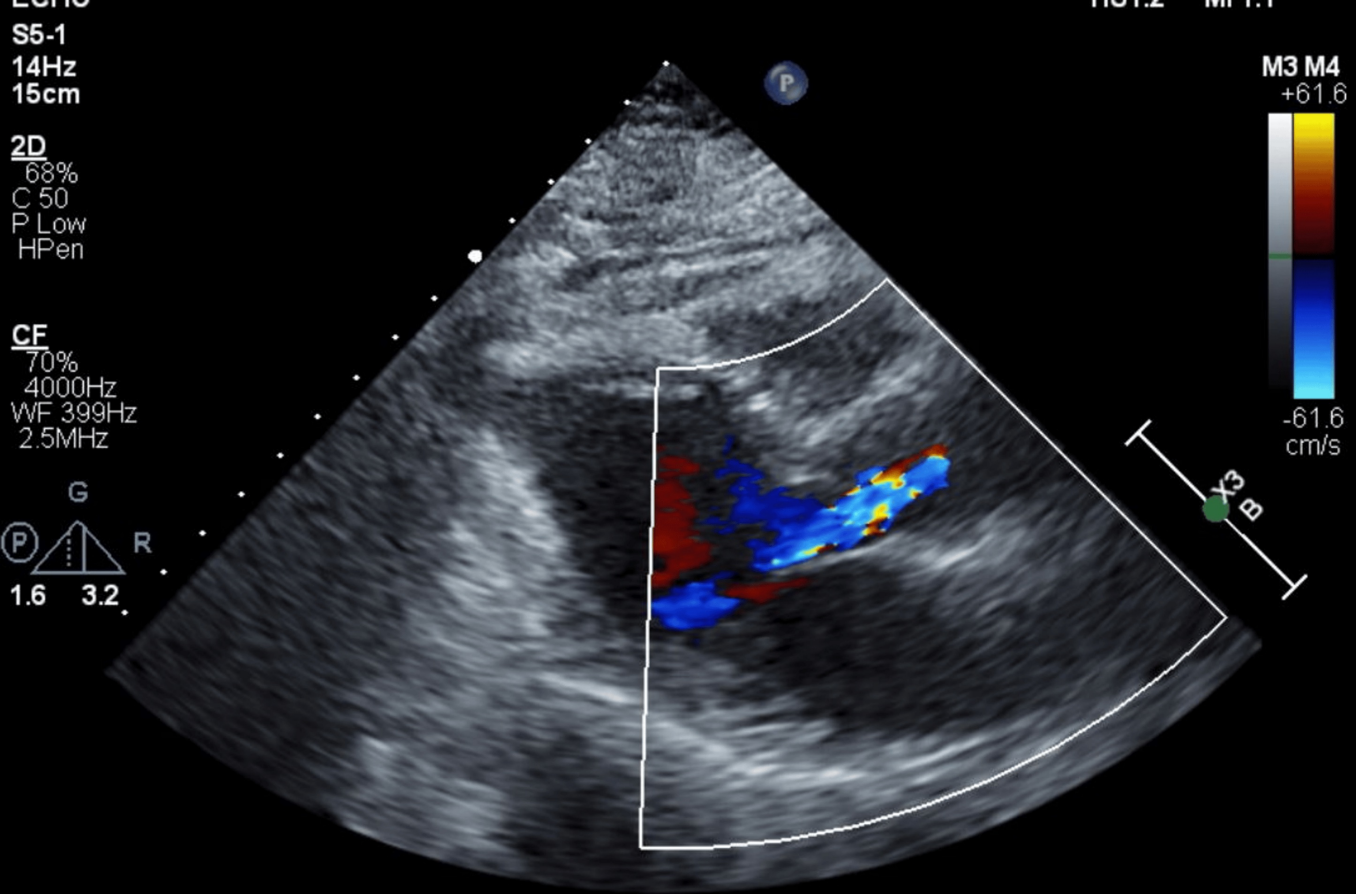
A transthoracic echocardiogram displaying a parasternal long-axis view with color Doppler showing mild-to-moderate aortic regurgitation
